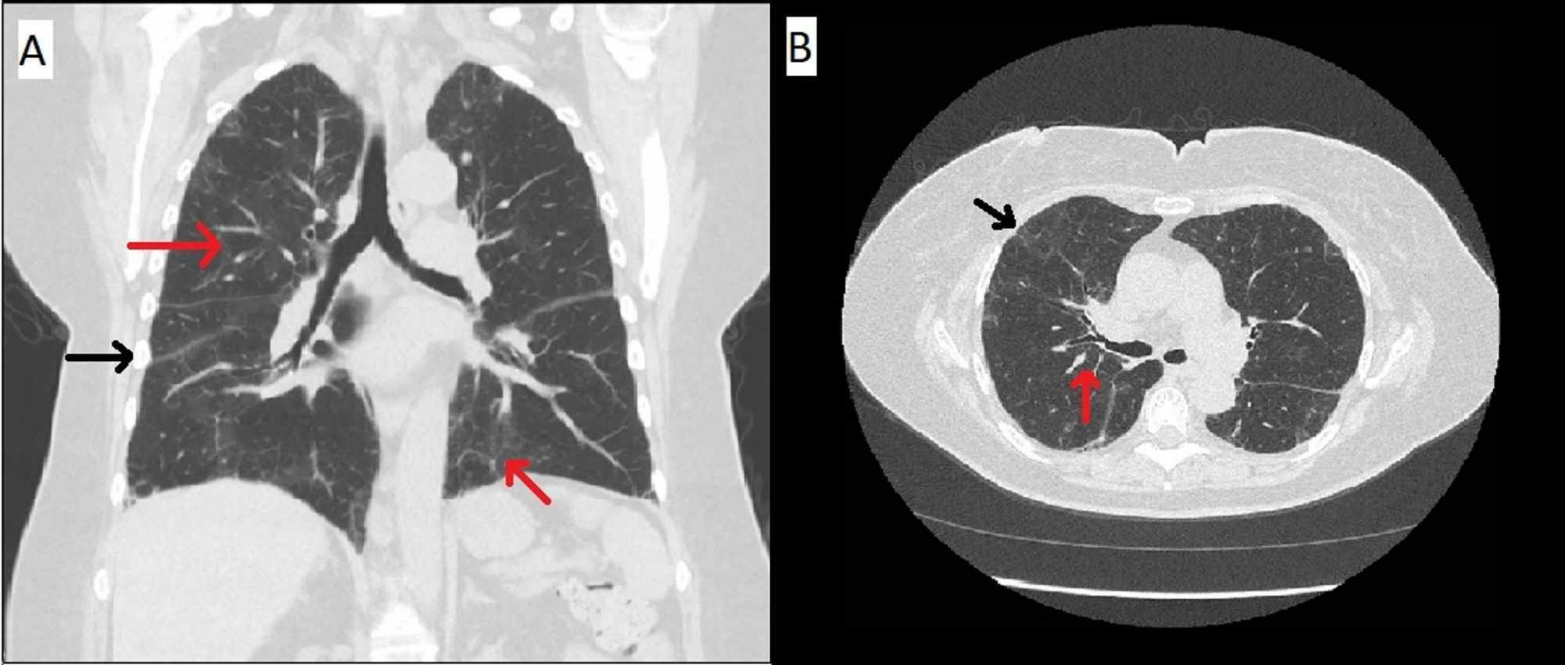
CT imaging three months after presentation Coronal CT chest imaging (A) and axial CT chest imaging (B) showing improvement of the upper lobe predominant peribronchovascular (red arrows) and subpleural nodular areas of consolidation and groundglass opacities (black arrows).
